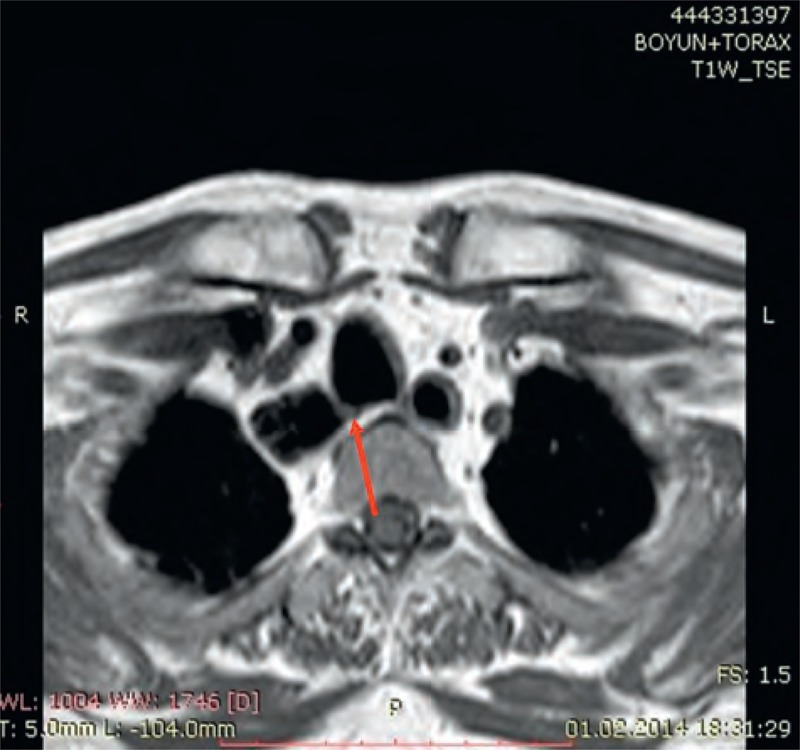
Thoracic magnetic resonance imaging revealed mediastinal cystic lesions communicating with the trachea (arrowhead indicates the fistula tract).
